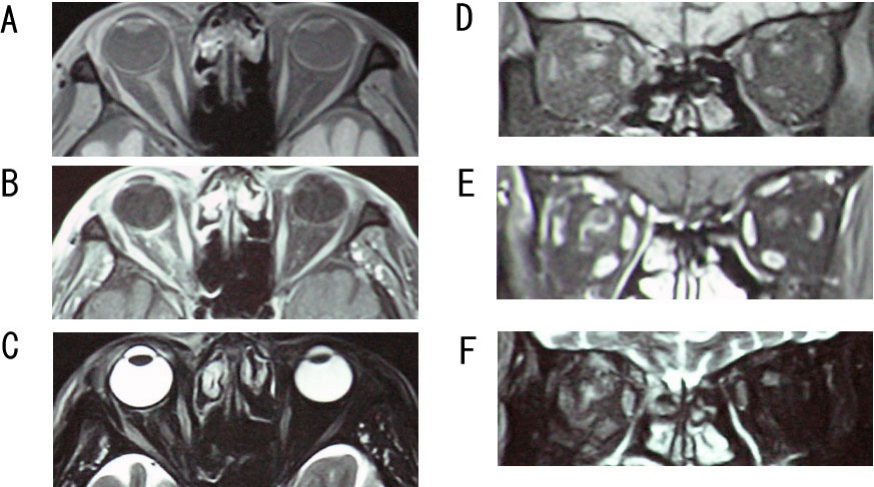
Images obtained by magnetic resonance imaging (MRI) in an eye with orbital apex syndrome. (A) Horizontal MRI, T_1_-weighted fat suppressed image. (B) Horizontal MRI, T_1_-weighted with Gadolinium enhanced image. (C) Horizontal MRI, T_2_-weighted and horizontal image. (D) Coronal MRI, T_1_-weighted fat suppressed image. (E). Coronal MRI, T_1_-weighted with Gadolinium enhanced image. (F) Coronal MRI, T2-weighted image. The right orbital cavity, external ocular muscles, and optic nerve are highly enhanced in the T_2_-weighted images and in the T_1_-weighted with Gadolinium enhanced images.
